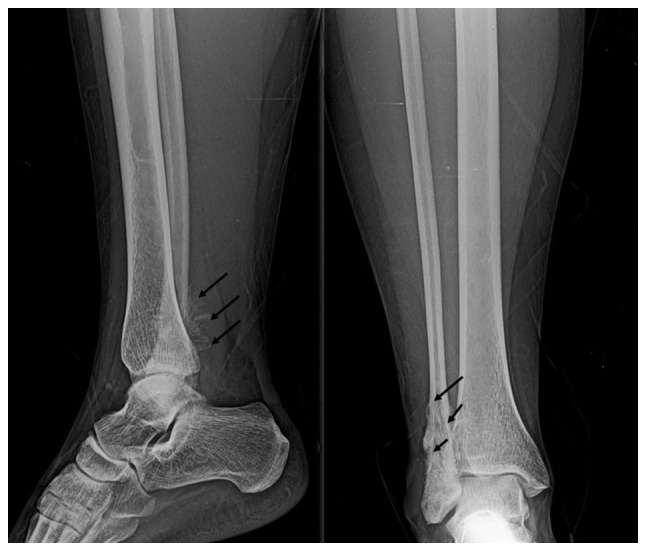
Anteroposterior and lateral ankle radiography: Shown are hypodense lesions located in the distal diaphysis of the right fibula with discontinuity of the cortex with the radiological sign of ‘rising sun’ (black arrows).
